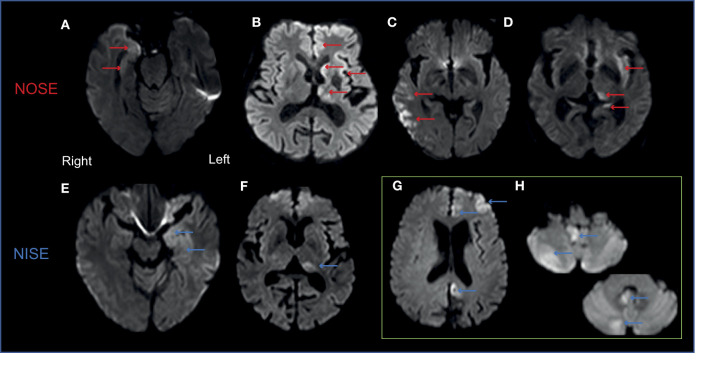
Generally similar patterns of PMA in NOSE and NISE. Examples of axial DWI views in 4 patients. (A) Right medial temporal hyperintensity in an 86-year-old woman with focal convulsive refractory NOSE. (B) Left thalamic, caudate nucleus, and frontal cortex hyperintensities in a 56-year-old man with generalized convulsive secondary non-convulsive super-refractory NOSE. (C) Right temporo-parietal cortex hyperintensity in a 79-year-old man with refractory generalized convulsive secondary non-convulsive NOSE. (D) Claustrum hyperintensity in the left hemisphere associated with a homolateral pulvinar and hippocampal hyperintensity in a 69-year-old alcoholic male with pharmacosensitive non-convulsive NOSE (SESA). (E) Left medial temporal hyperintensity in a 48-year-old man with pharmacosensitive focal non-convulsive NISE. (F) Left pulvinar hyperintensity in a 70-year-old man with pharmacosensitive focal non-convulsive NISE. (G, H) Right cerebellum hyperintensity and contralateral cortical hyperintensity in a 63-year-old man with a non-convulsive super-refractory NISE.
